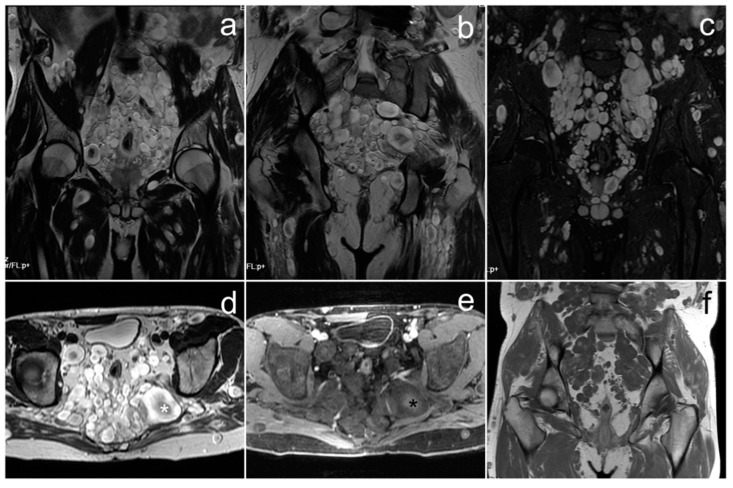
Pelvic MRI in Case 1 (a–f). In images (d,e), typical signs of neurofibroma are marked with asterisks: (d)—”target sign”; (e)—”reverse target sign”. (a,b,d)—FSE/T2; (c)—STIR; (e)—post-contrast FSE/T1 F; (f)—post-contrast FSE/T1 post-Gd.
